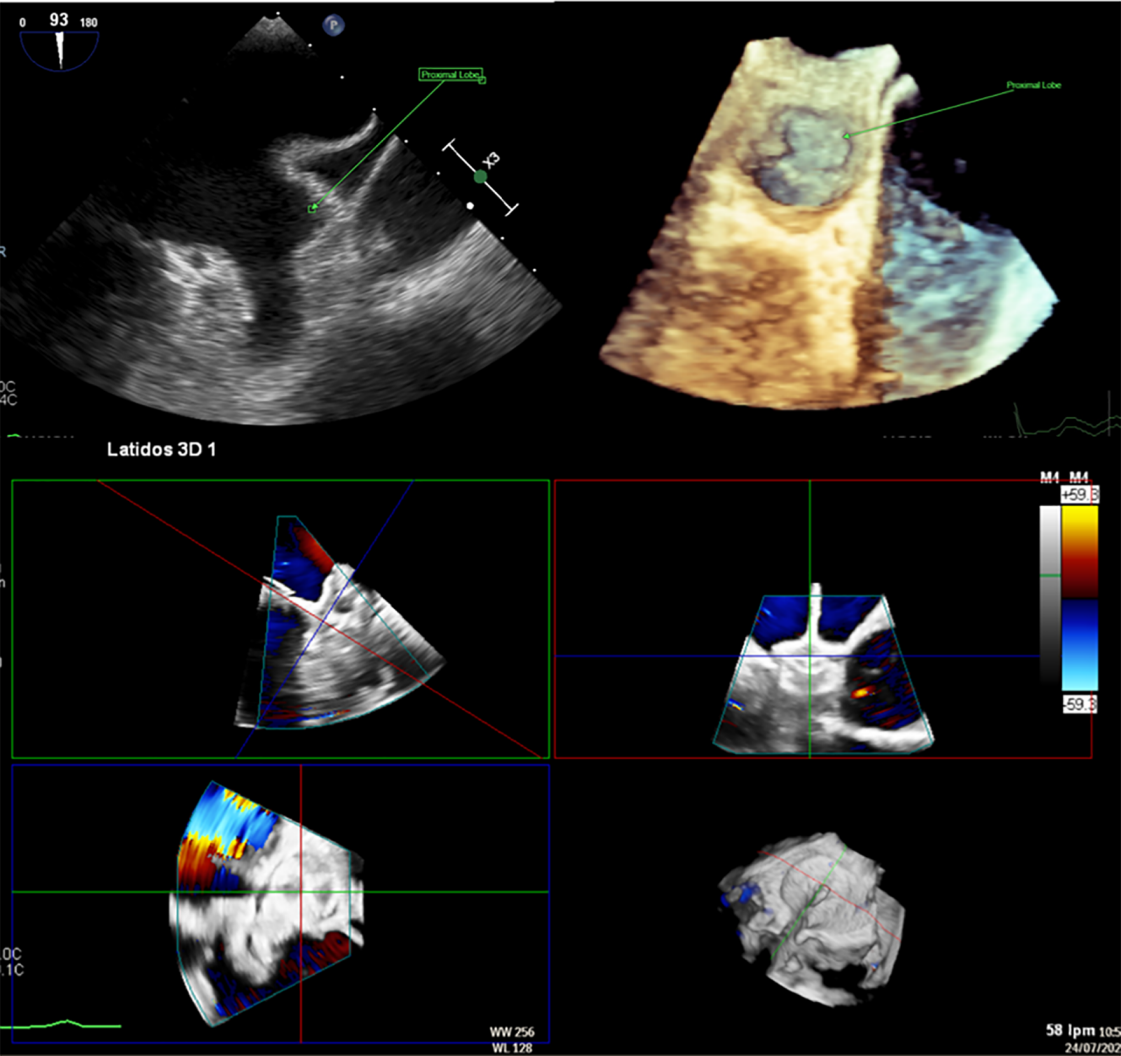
Left atrial appendage closure (LAAC) in a patient with complex anatomy. Transesophageal echocardiography (TEE) revealed a left atrial appendage (LAA) with a large proximal lobe, presenting a
